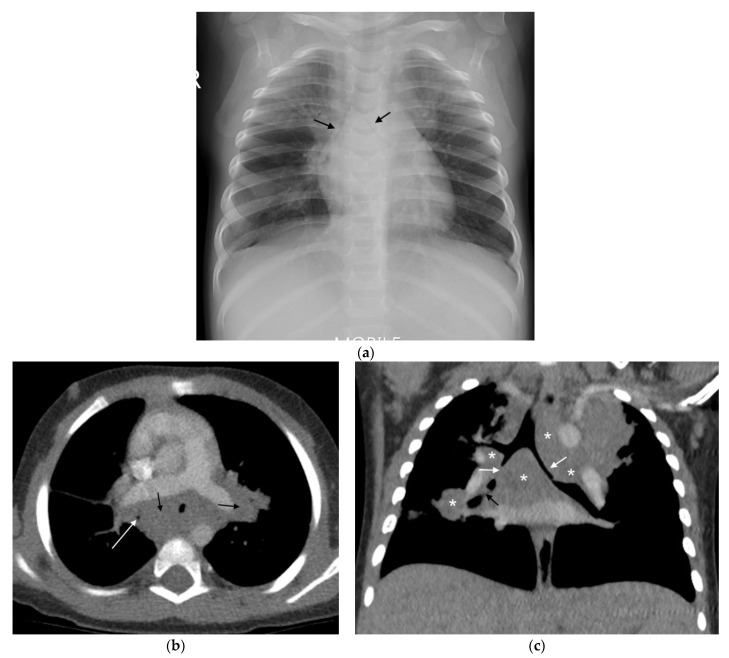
(a–c): Lymphobronchial TB. Chest radiograph and CT in a 13-month-old boy with confirmed pulmonary TB: (a) The frontal AP chest radiograph is suggestive of bilateral hilar and paratracheal lymphadenopathy by the presence of bronchus intermedius and left main bronchus compressions resulting in bilateral (black arrows), mid and lower zone air-trapping. (b) Axial, post-contrast, soft-tissue-windowed CT scan at the level of the pulmonary trunk bifurcation demonstrates extensive subcarinal and hilar lymphadenopathy (black arrows) with marked bronchus intermedius attenuation (white arrow). (c) Coronal reconstruction of the post-contrast soft-tissue-windowed CT scan demonstrates paratracheal, sub-carinal and hilar lymphadenopathy (stars). There is attenuation of the bronchus intermedius and the left main bronchus (white arrows). There is also a suggestion of erosion of a right hilar lymph node into the lumen of the bronchus intermedius (black arrow).
